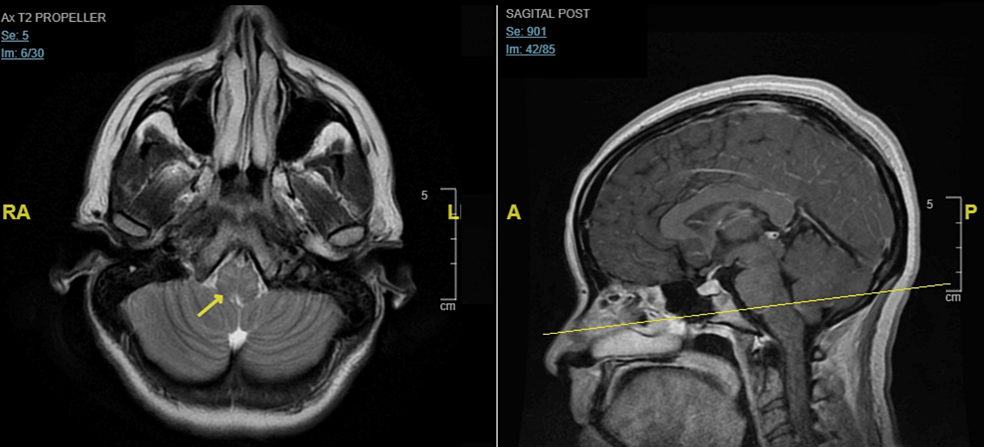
MRI brain axial T2 (right) and sagittal (left) post-contrast section
